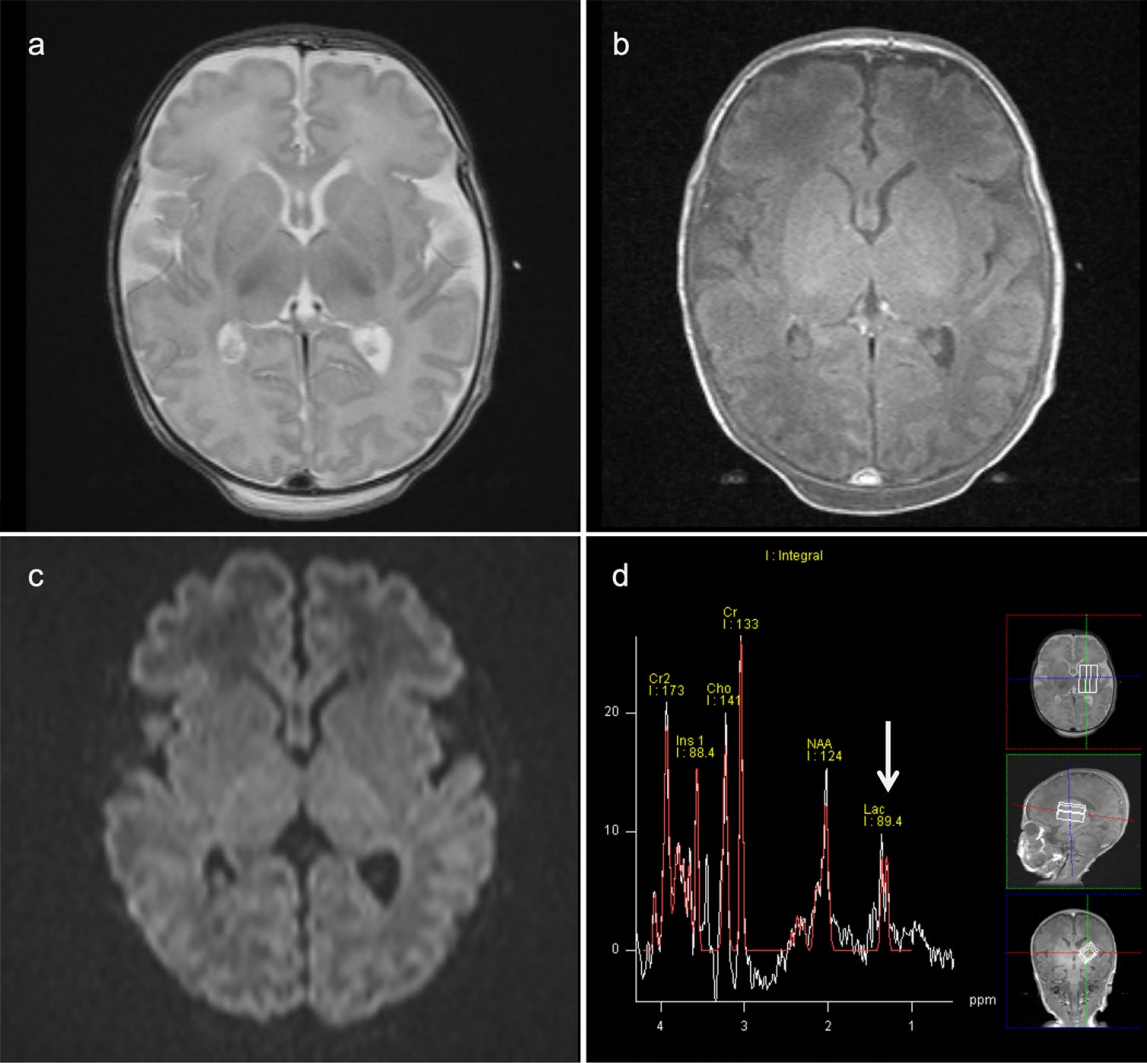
An encephalopathic neonate with methylmalonic acidemia (MMA). a, b T2- (a) and T1-weighted images (b) indicated diffuse T1 and T2 prolongations in the cerebral white matter. c No restricted diffusion was visible, but the white matter showed a relatively a low DWI signal. d
